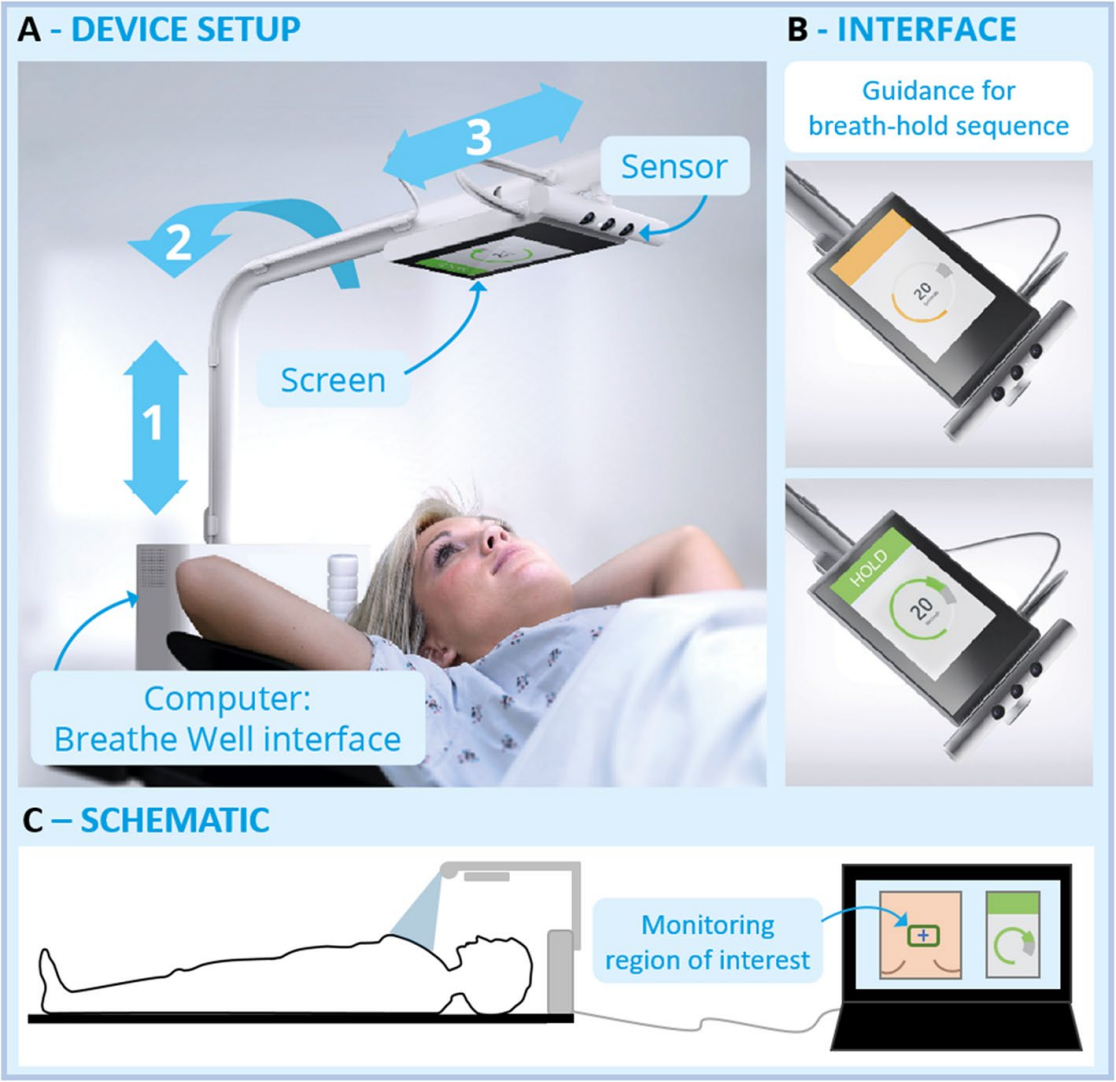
The Breathe Well device. A Similar setup on the CT and treatment couch. B Patient screen with breath hold position visualisation and instructions. C Optical feed and breath-hold depth monitoring central chest area displayed to the radiation therapist
